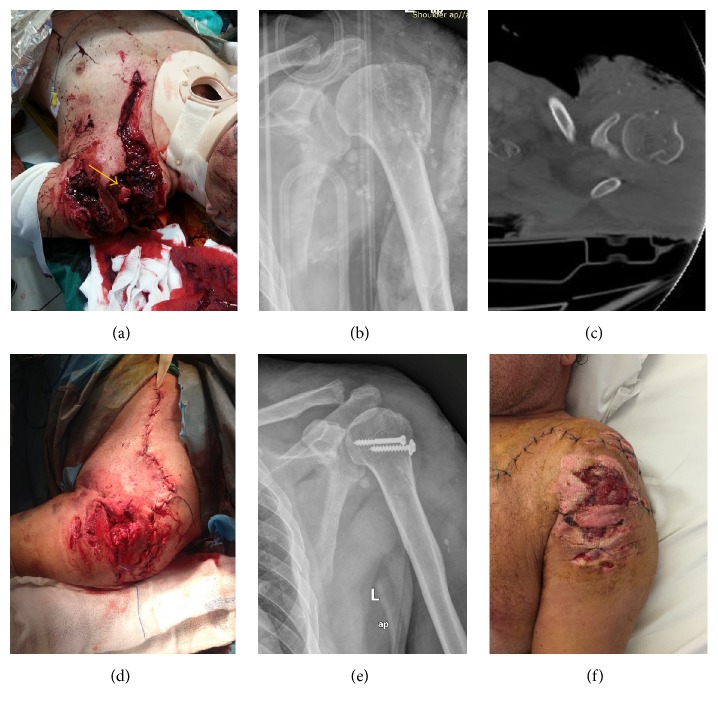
(a) Photography of the trauma during patient resuscitation indicated severe, sword-like injury to the left shoulder, with open fracture of the humeral head (arrow). (b) Preoperative anteroposterior X-ray of the left shoulder showing head-splitting fracture of the proximal humerus and presence of multiple foreign bodies (glass). (c) CT scan of the left shoulder indicating involvement of ~30% of the articular surface and the greater tuberosity. (d) Intraoperative picture after muscle and skin closure. (e) Postoperative X-ray of the left shoulder showing adequate reduction of the fragment. (f) Condition of the skin at the 10th postoperative day just before a split skin graft was about to apply.
